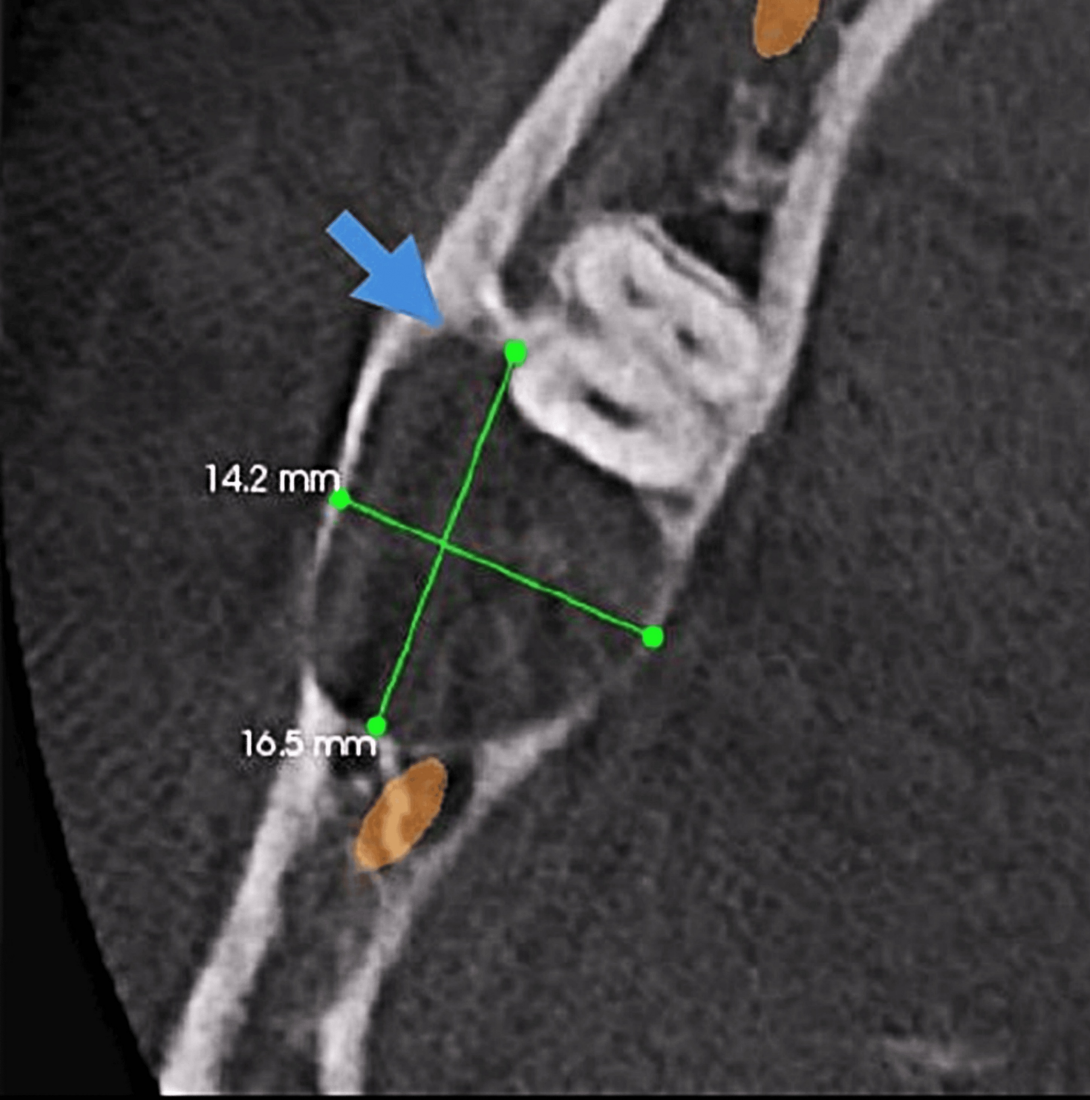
Axial view of CBCT revealed a well-defined solitary radiolucent lesion of approximately 16.5 × 20.7 × 14.2 mm (AP × SI × BL) with respect to the right posterior mandibular anterior ramus region, extending anteroposteriorly from CEJ of 48 to distal aspect of 47, and superoinferiorly from crestal bone to approximately 8.8 mm from inferior border of mandible. CBCT: Cone beam computed tomography; AP: Anteroposterior; SI: Superoinferior; BL: Buccolingual; CEJ: Cementoenamel junction
